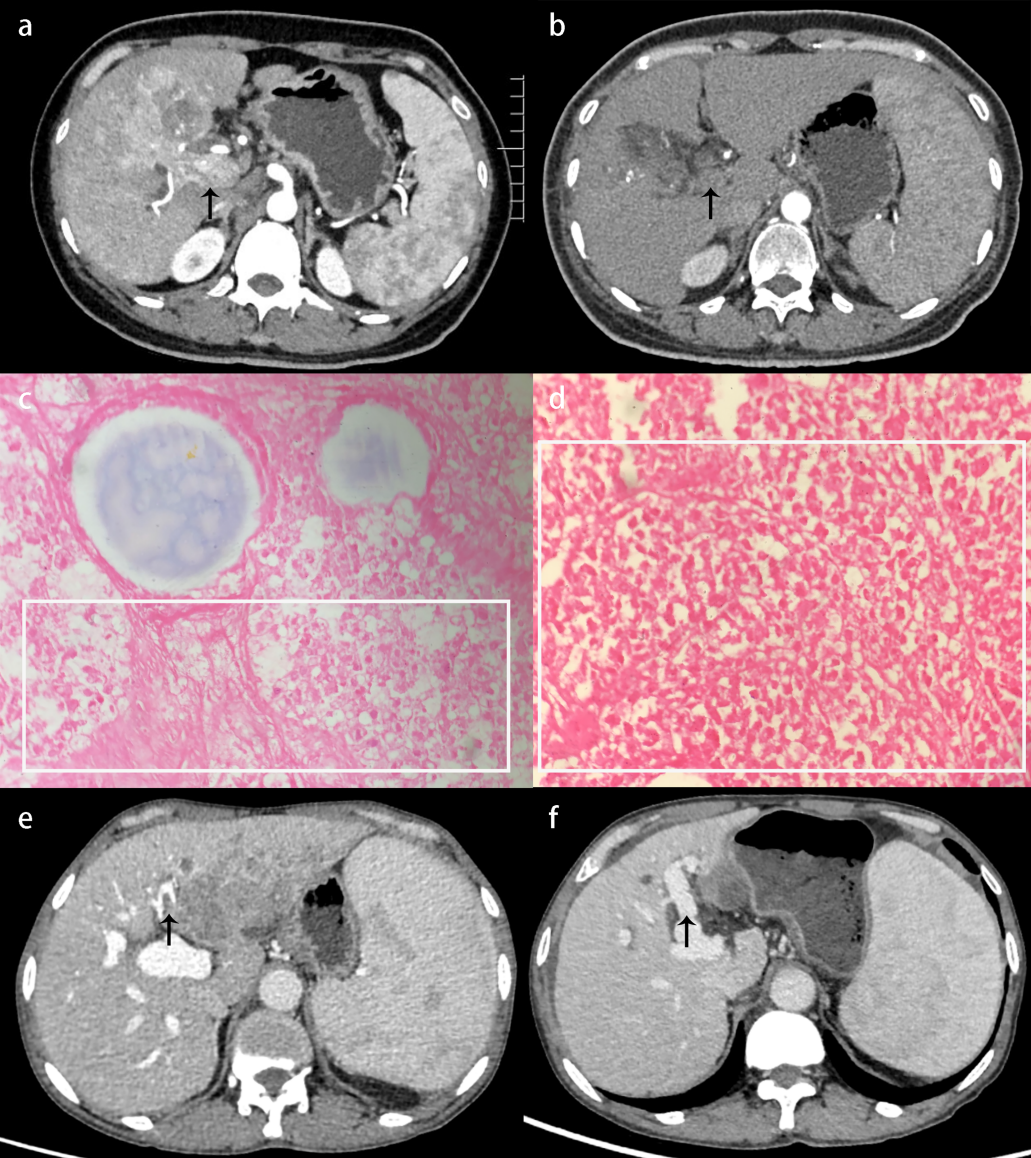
Presentation of two downstaged patients with HCC. (A) Before administering the combination therapy, contrast-enhanced CT exhibited tumor thrombus in the main trunk of the portal vein with enhancement during the arterial phase (black arrow). (B) After 4.5 months of the combination therapy, contrast-enhanced CT exhibited shrunken tumor size and necrotic tumor tissues in the portal vein without enhancement (black arrow). (C) Pathological examination confirmed HCC with massive necrosis (×400); the necrotic region is shown in a white rectangle. (D) Pathological examination confirmed that the PVTT was completely necrotic (×400); the necrotic region is shown in a white rectangle. (E) Contrast-enhanced CT showed tumor thrombus in the left main trunk of the portal vein with enhancement during the portal venous phase (black arrow). (F) After 6.5 months of the combination therapy, contrast-enhanced CT showed shrunken tumor size and absence of PVTT (black arrow). CT, Computed tomography; HCC, hepatocellular carcinoma; PVTT, portal vein tumor thrombus.
